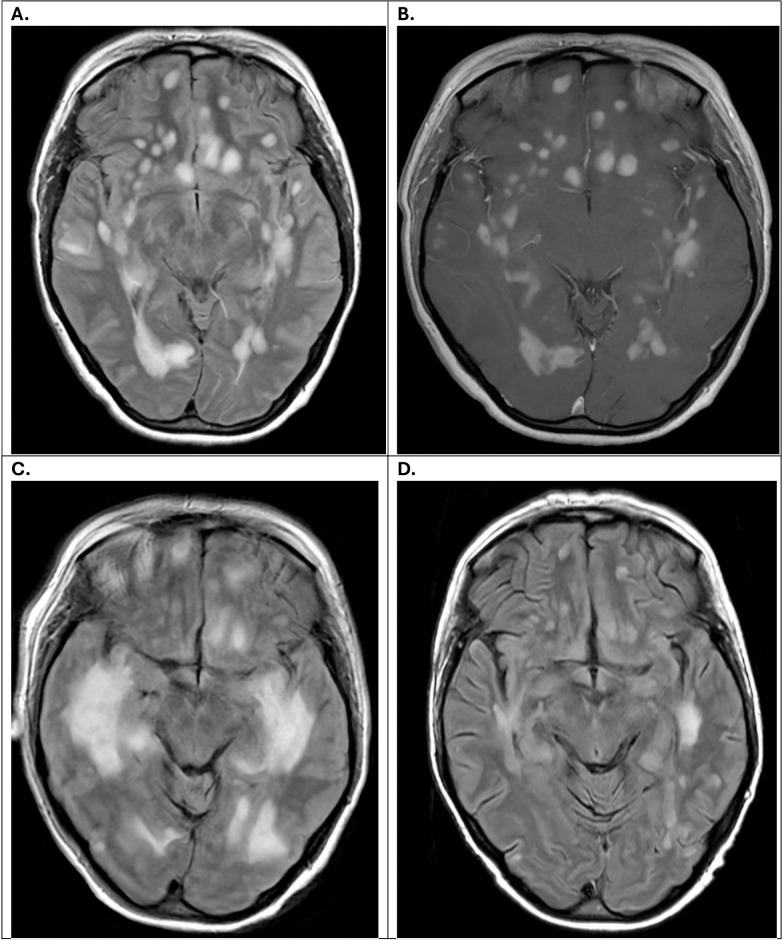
MRI brain images at initial presentation (A, B), post 5 days of high dose steroids, plasmapheresis X 5 sessions and IVIg X 5 days (C), post HiCy X 2 doses (D). (A) Initial MRI brain axial T2 FLAIR image showing extensive supratentorial lesions. (B) Initial MRI brain axial T1 post contrast image showing extensive demyelination along the supratentorial lesions. (C) MRI brain axial T2 FLAIR image post high dose IV steroids for 5 days, plasmapheresis X 5 sessions, and IVIg X 5 sessions showing progression of the lesions with increased size of the supratentorial lesions. (D) MRI brain axial T2 FLAIR section post HiCy X 2 doses showing stabilization of the supratentorial lesions with decrement of the size.
